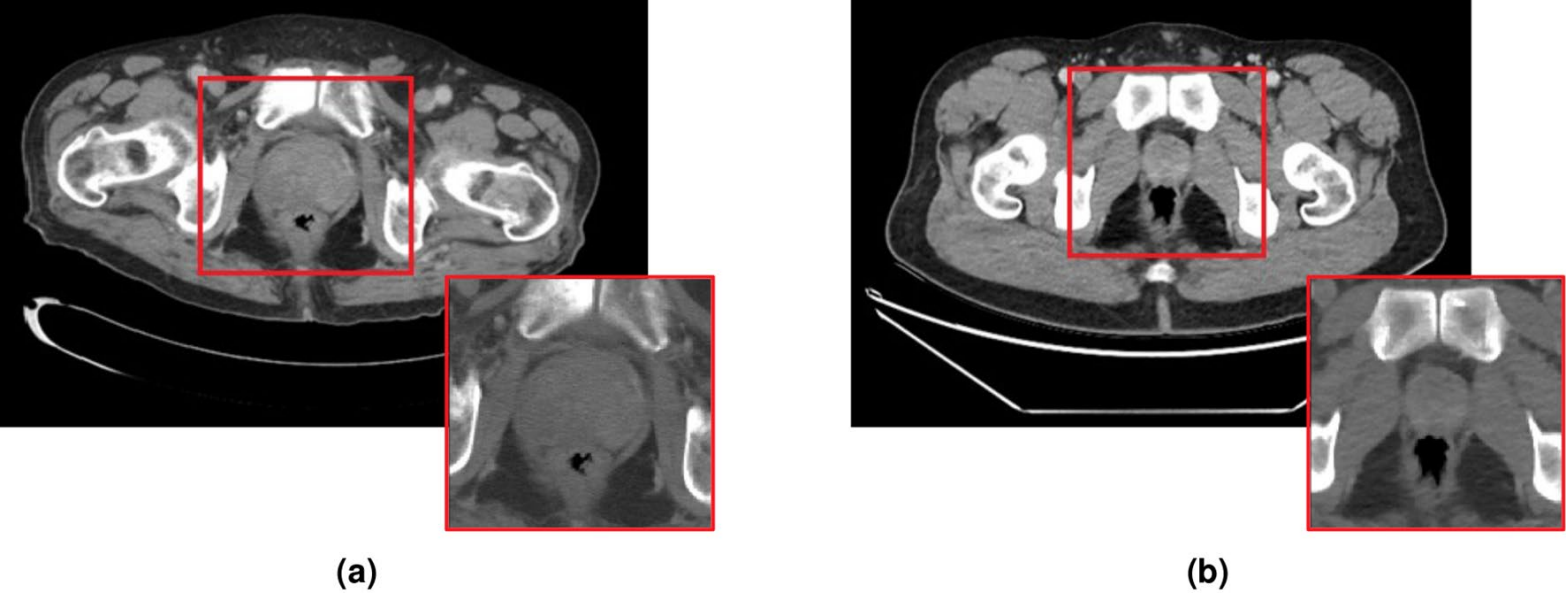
Two CT scan slices from the dataset: (a) from a confirmed csPCa patient, and (b) from a patient with no known prostate cancer (control). Both include a red square indicating the cropped prostate region, and the pre-processed version on the lower right corner.
